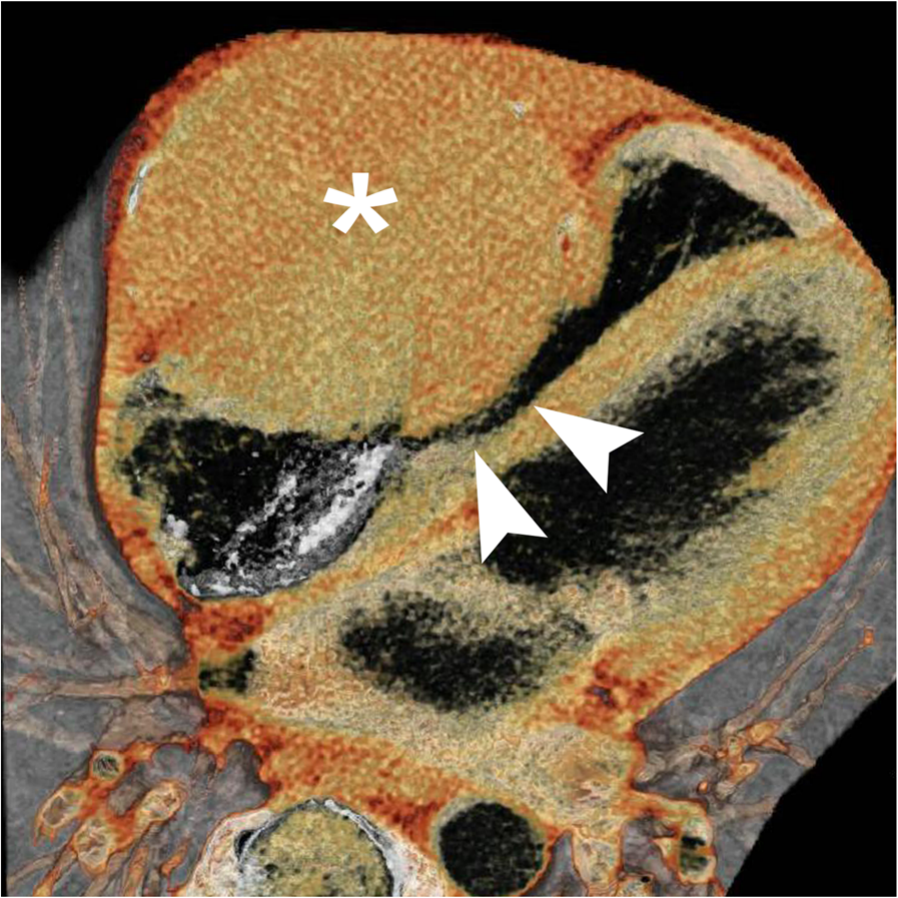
CT Reconstruction of Aneurysm - Blood-pool inversion volume rendered 4-chamber image shows the extent of luminal compression and restriction in diastolic filling of the RV and tricuspid areas (arrowheads) by the mass (*).
